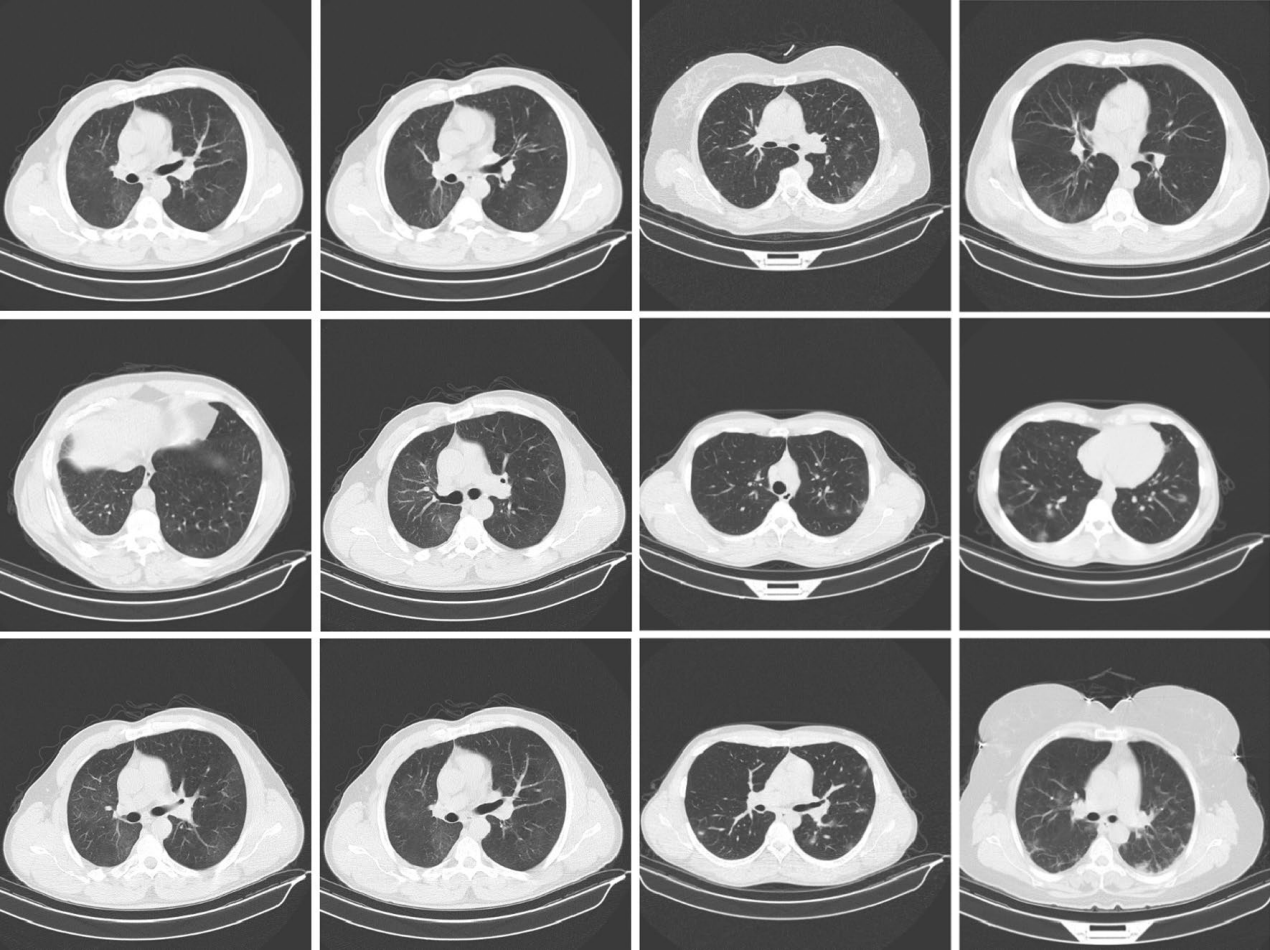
Sample lung CT scan images from dataset 1.
